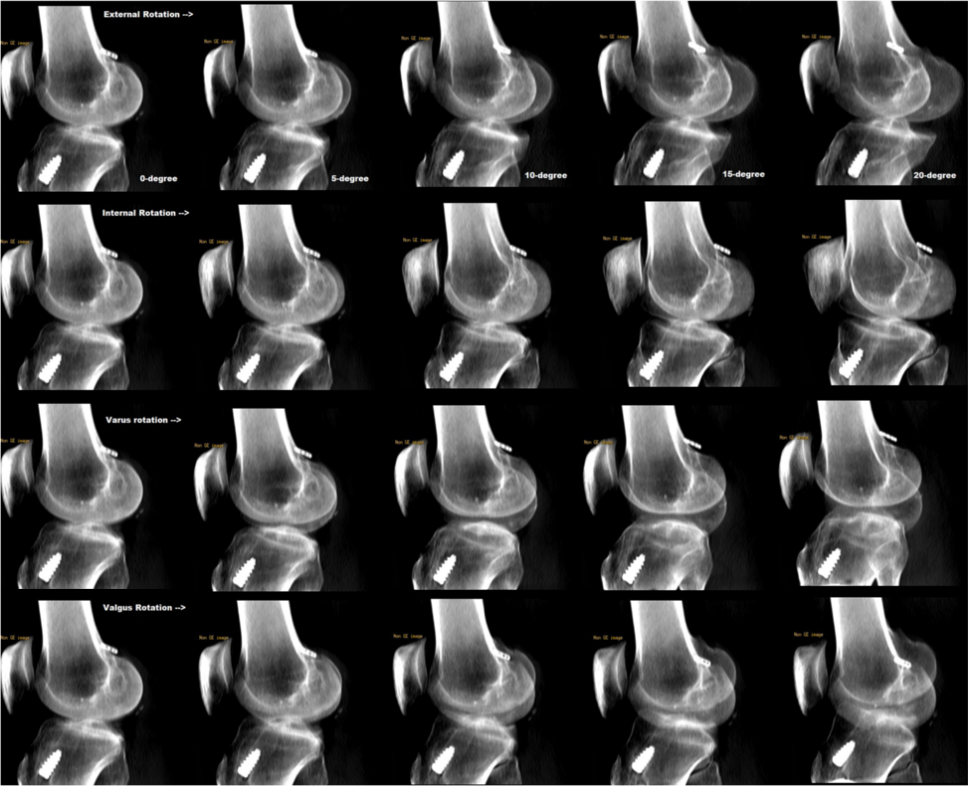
Thumbnails of digitally reconstructed radiographs of a representative case in 0 to 20-degree of external, internal, varus and valgus rotations. 0-degree rotation represents a true lateral radiograph
